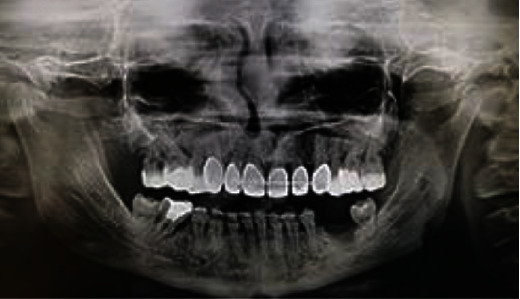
Panoramic radiograph on a follow-up appointment after 24 months.
